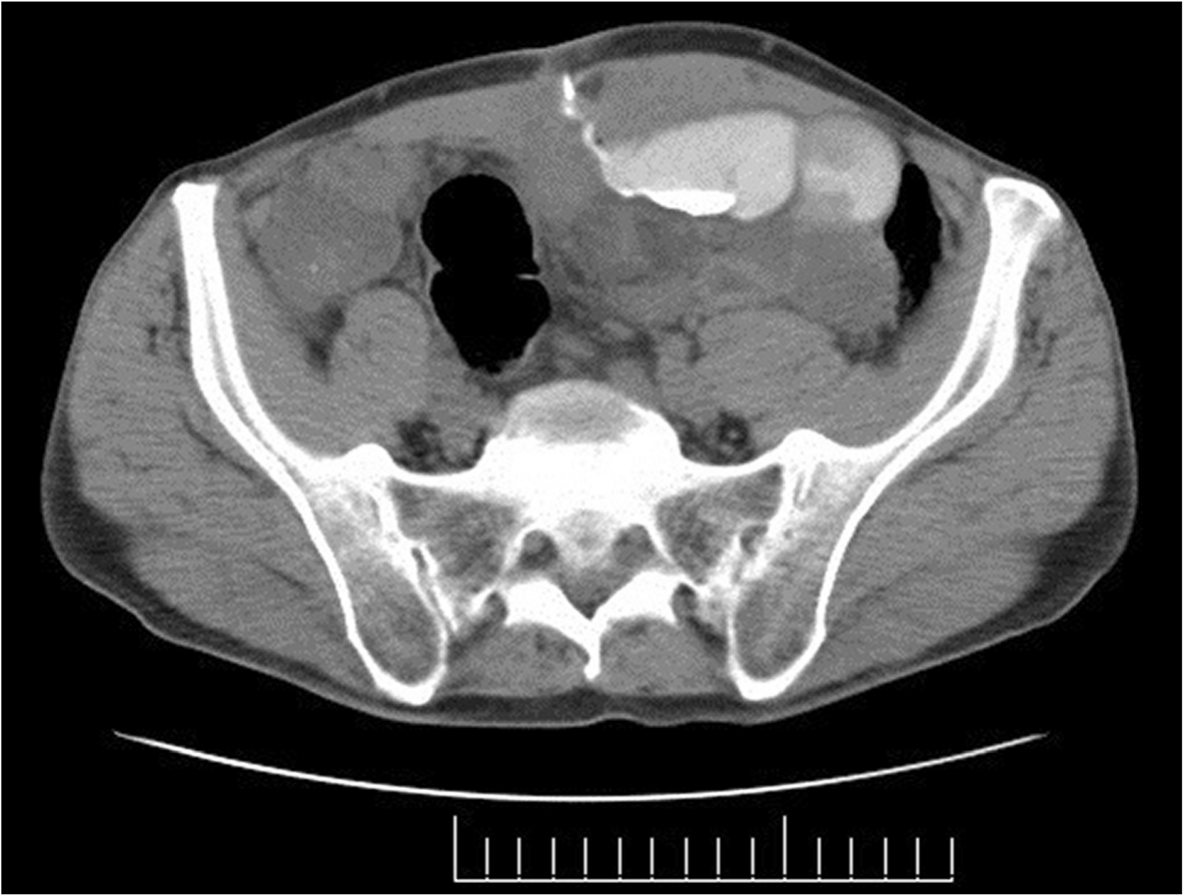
Patient CT fistulogram 2008.
